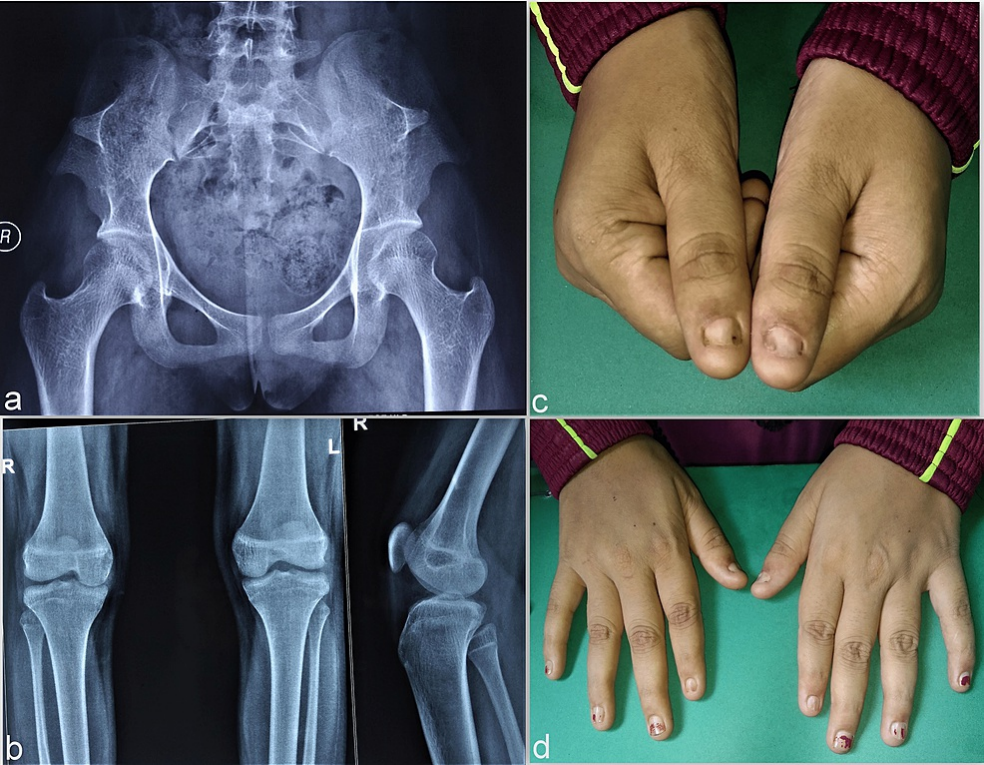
Clinical and radiological features of Case 1 The radiographs showing the presence of bilateral iliac horns in the pelvic region (a). Lateral knee radiograph showing smaller-sized patellae (b). The presence of the hypoplastic changes is more pronounced on bilateral thumbnails (c). Other fingernails show varying grades of hypoplasia and dystrophy (d).
